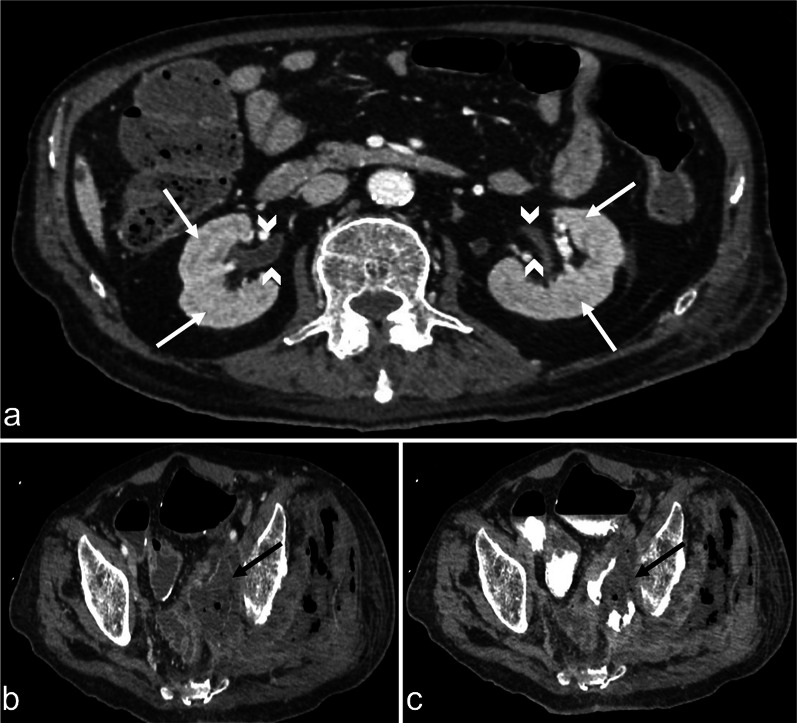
Contrast-enhanced CT images in the portal venous phase (a and b) and the three-minute-delayed phase (c), showing a patient with septic shock (qSOFA 2) due to entero-neovesical fistula (b and c, black arrows) with pyelitis (a, arrowheads) after radical cystectomy. The decreased enhancement of the renal medulla (a, white arrows) in the venous phase was observed
